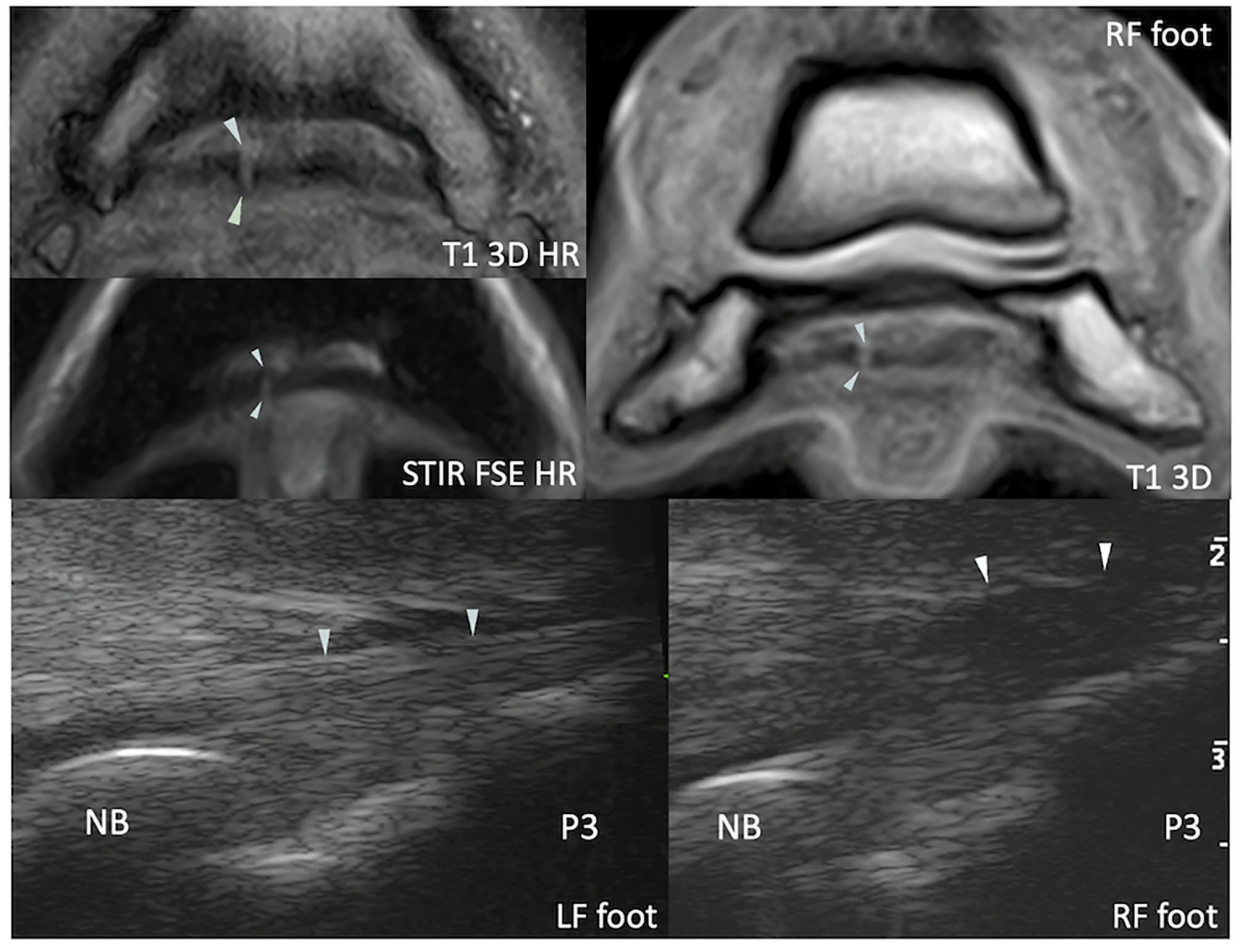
Transverse magnetic resonance (MR) images of the right front foot (top) and comparative transcuneal longitudinal ultrasonographic images of the left and right front feet (bottom) at the infrasesamoidean level. A hyperintense parasagittal tendon split is visible in the medial lobe of the deep digital flexor tendon on MR images (arrowheads). At ultrasound, the abnormal tendon (right front foot) appears thickened in its most distal portion and has a slightly convex palmar border (arrowheads). The tendon also appears hypoechoic in comparison to the contralateral foot, although this may be due to anisotropy and induced by a non-parallel fiber alignment to the ultrasound probe. T1 3D (HR), (high resolution) tridimensional gradient echo T1 weighted image; STIR FSE HR, high resolution fast spin echo short tau inversion recovery weighted sequence; NB, navicular bone; P3, distal phalanx.
